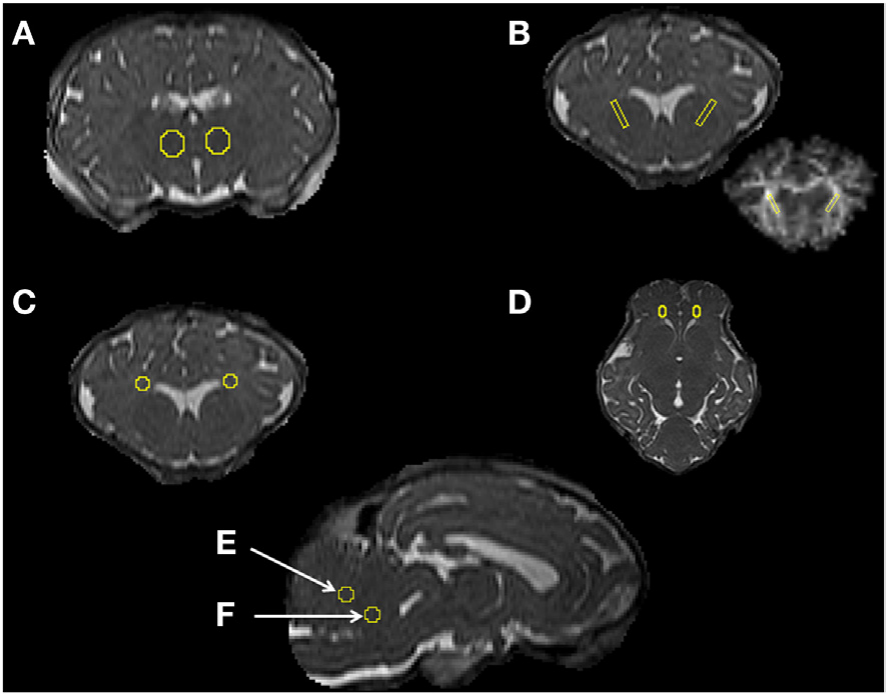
Magnetic resonance imaging data analysis [regions of interest (ROIs)]. Examples of ROIs in specific regions of the thalamus (A), internal capsule (B), periventricular white matter (C), frontal white matter (D), and the cerebellum vermis, targeting the midline and stalk white matter (E,F) for DTI analysis.
